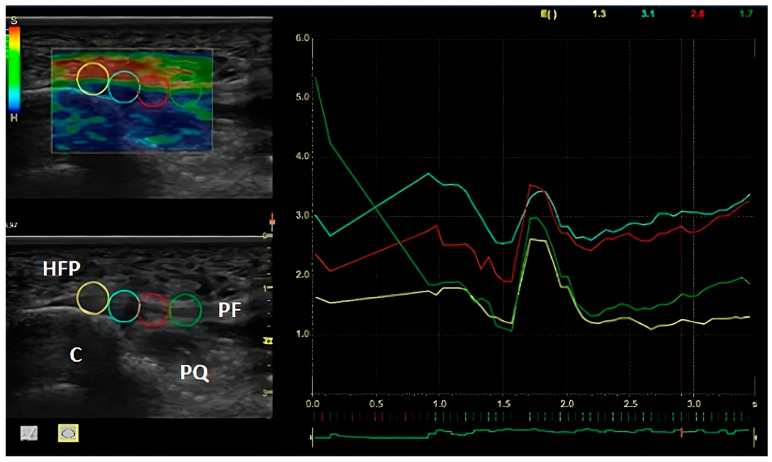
SEL measurements. Region of interest (ROI) and SEL measurements. Note: Left to right: Point 1: PF insertion to the calcaneus; Point 2: body of the FP in mid-proximal portion; Point 3: body of the FP in middle portion; Point 4: body of the PF in mid-distal portion. Note: The anatomic structures on the ultrasound imaging: HFP (heel fat plantar), PF (plantar fascia), C (calcaneus), PQ (plantar quadratus).
